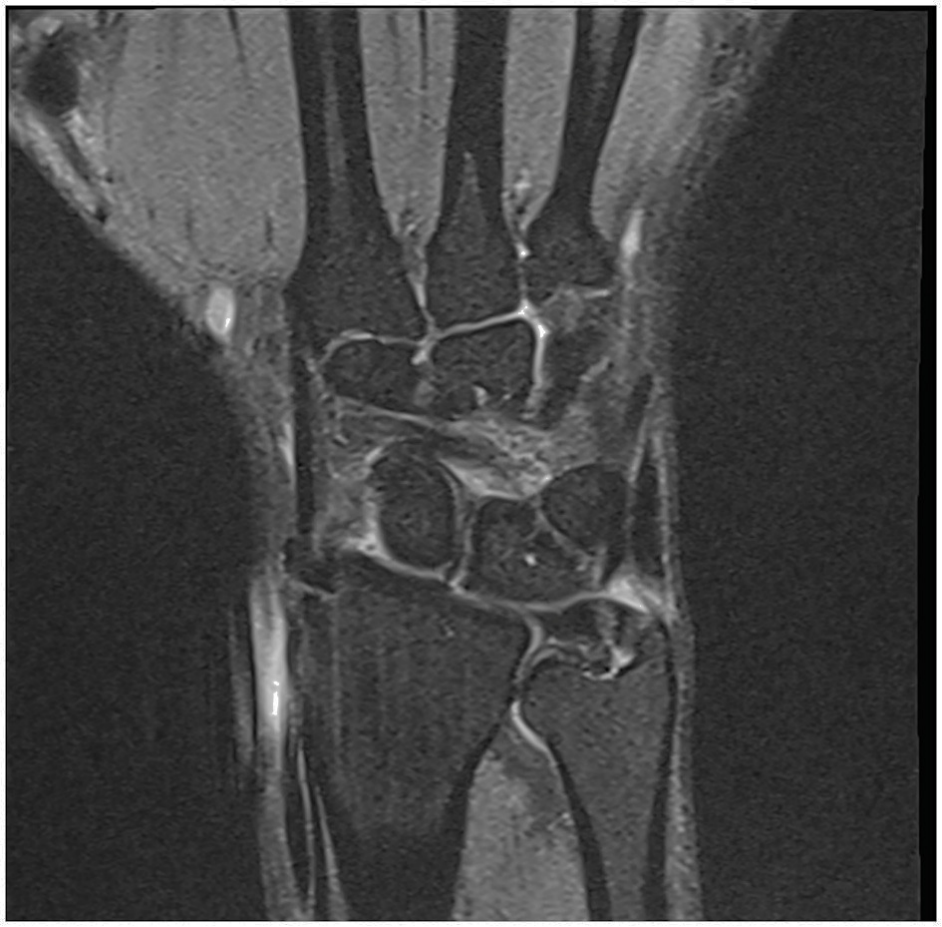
Coronal MRI of the right wrist demonstrating a tear in the triangular fibrocartilage.
